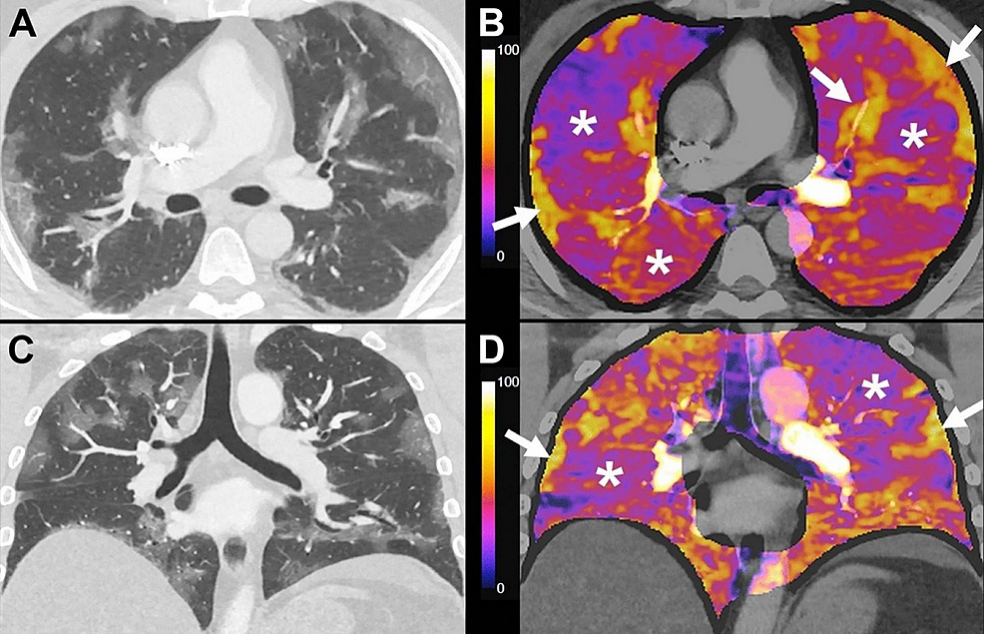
Perfusion CT imaging of a COVID-19 lung reveals areas of hyper and hypoperfusion The CT scan is of the lungs of a 37-year-old male patient with RT-PCR-confirmed COVID-19 and was taken 10 days post symptom onset. Admission PaO2/FiO2 ratio was 240 and the d-dimer level was 480 ng/mL. The patient was admitted to the ICU and managed with invasive mechanical ventilation. A, C: Axial and coronal lung-window chest CT angiography images show multiple foci of ground-glass opacities, with a predominantly subpleural distribution, with areas of apparently healthy lung parenchyma. Vascular dilatation can be seen in areas of ground-glass opacities. B, D: 5 mm reconstruction images of subtraction iodine maps in corresponding axial and coronal planes, show areas of severe hypoperfusion in the corresponding healthy lung parenchyma (*), with increased perfusion in areas of ground-glass opacities (white arrows). Figure inserted with permission from publisher [12].
